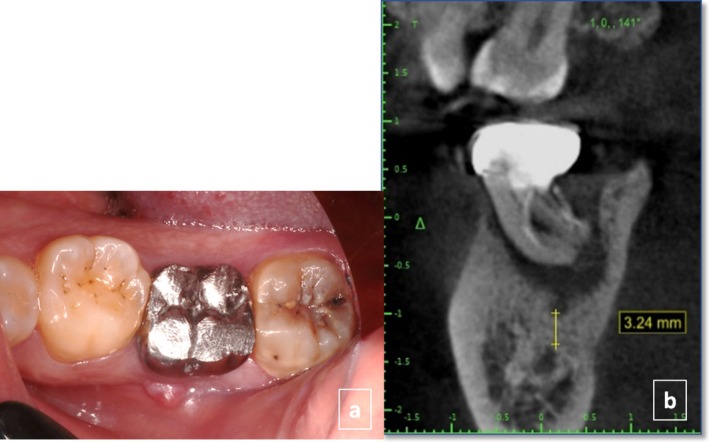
(a) Preoperative clinical view of the second mandibular right molar showing a chronic abscess; (b) CBCT coronal view of the large periapical lesion with significant alveolar bone resorption. A vertical fracture is visible on the distal root.
